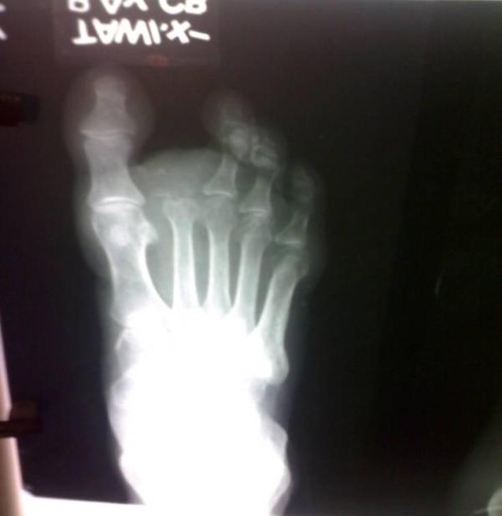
Postoperative radiograph of the foot.
